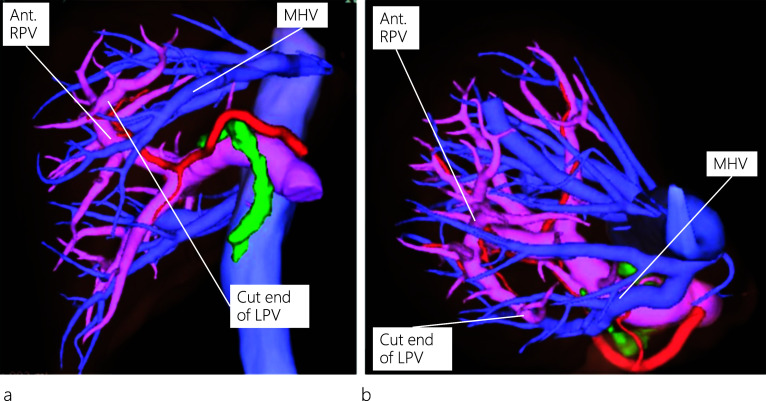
Three-dimensional reconstruction of postoperative enhanced CT images. Both the MHV and Ant. RPV are preserved, as viewed from neutral (a) and left posterior oblique (b) positions. CT: computed tomography; MHV: middle hepatic vein; Ant. RPV: anterior right portal vein; LPV: left portal vein
